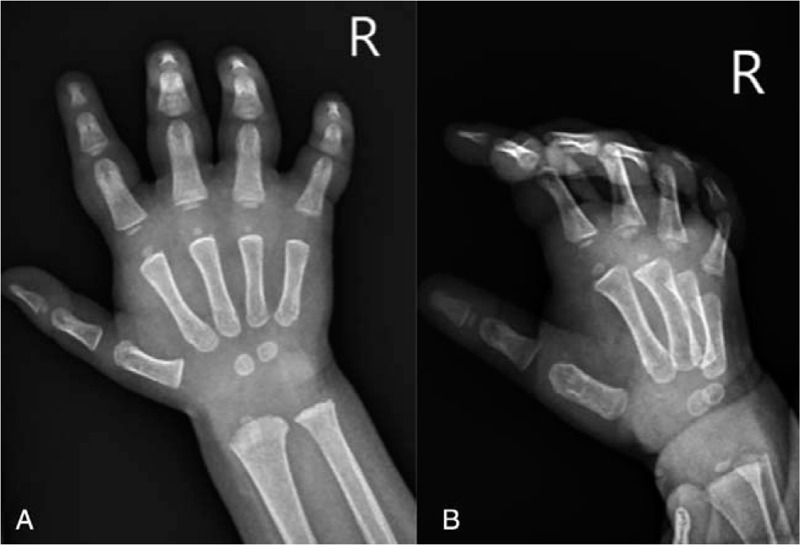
(A) Radiographic AP-image, (B) radiographic lateral-image.
